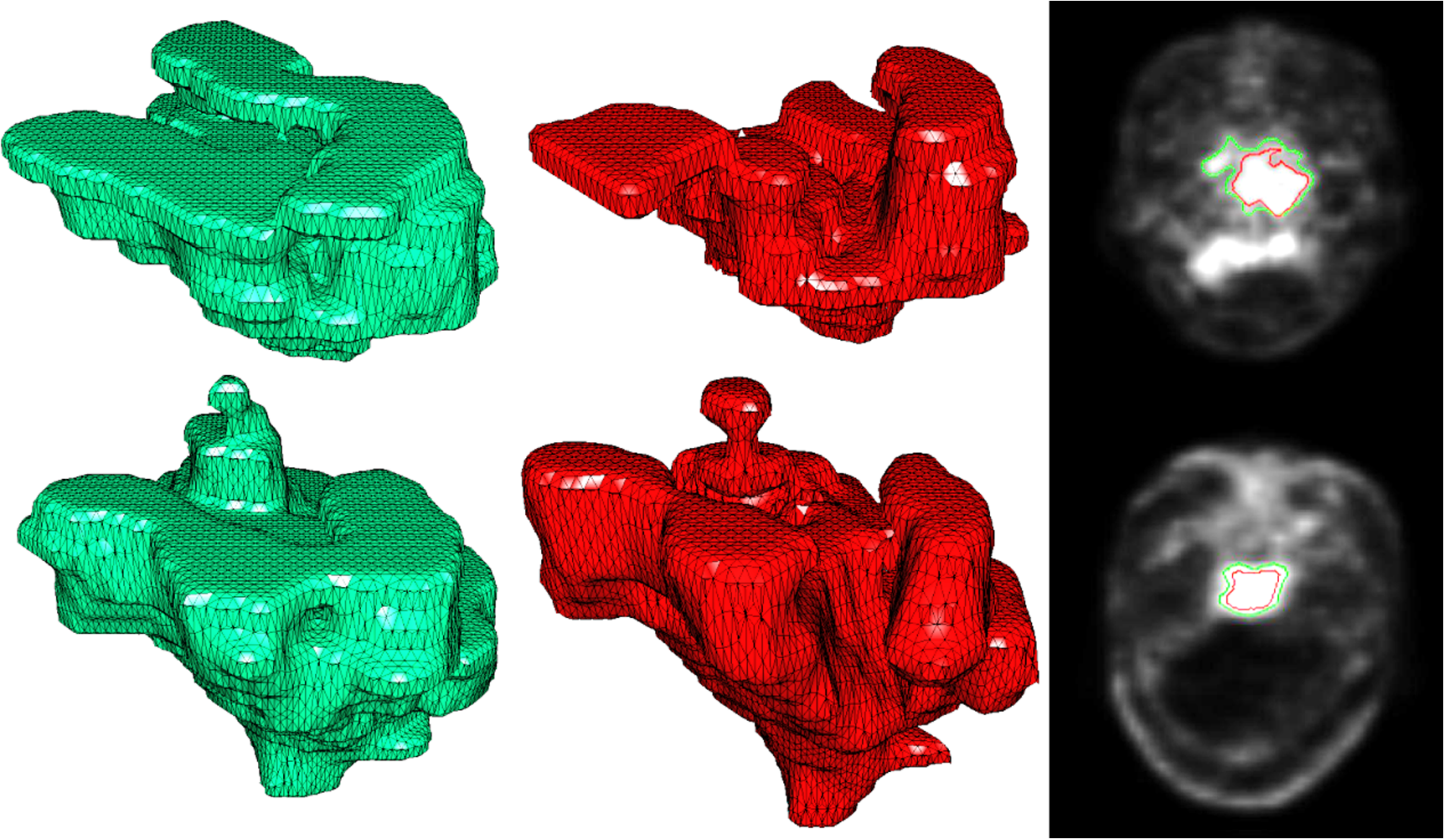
Solid rendering of manually contoured reference (green) and RG result (red). The first row shows the result of ^18^F-FDG; the second row shows the result of ^11^C-Choline. The DSCs of the two modalities were 67.06% and 74.11%, respectively. (^11^C-Choline scans were obtained 3 d after ^18^F-FDG).
